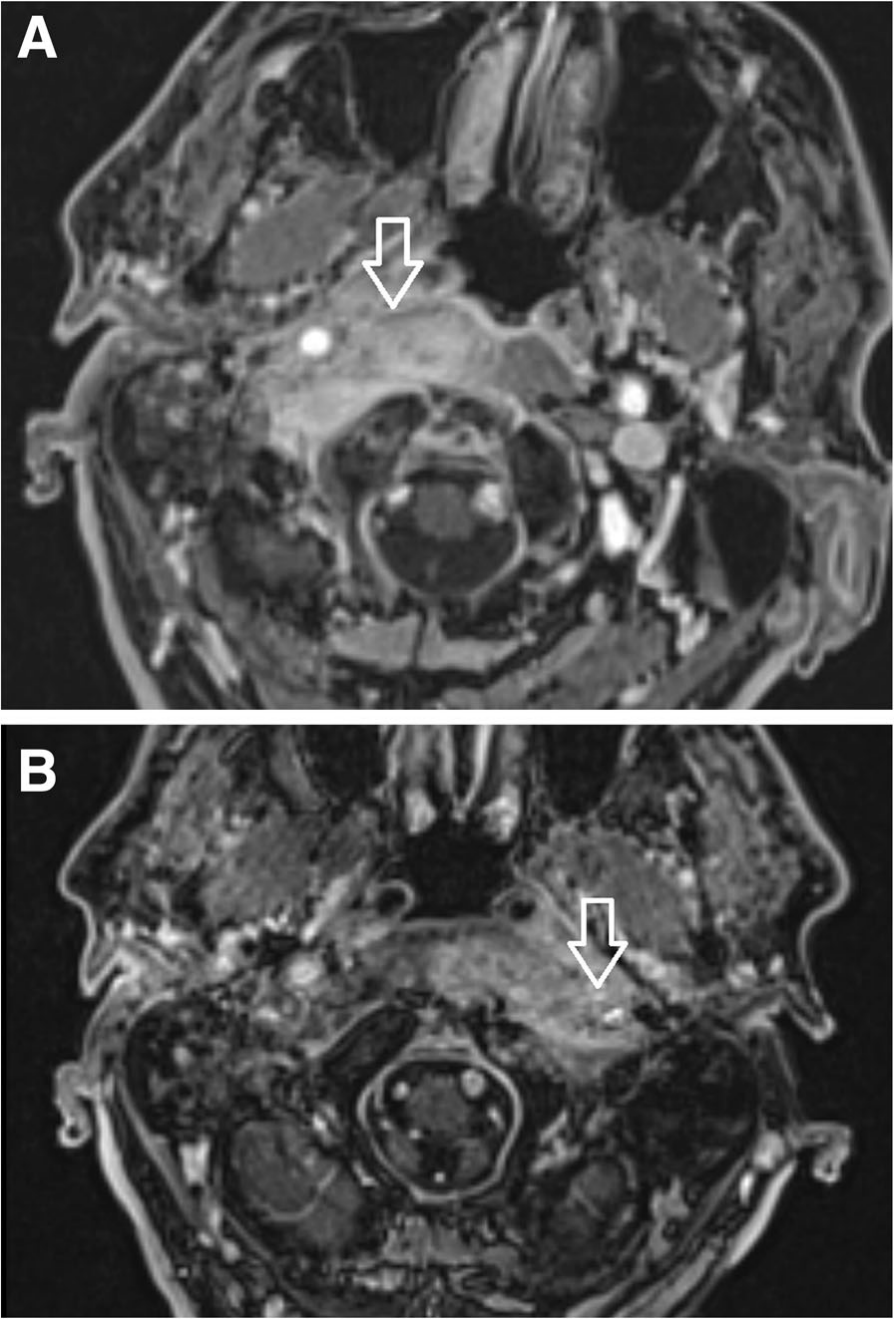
a Patient 1, 2 months after the first clinical symptoms: MRI of the skull base. Contrast-enhanced axial T1-weighted spin-echo image with fat saturation. Inflammatory changes of the right side soft tissues beneath the skull base, musculus longus capitis and musculus rectus capitis anterior (arrow). The swelling extends to the midline; the infection originates from the right external auditory canal. b Patient No. 1, 4 months following the first clinical symptoms: MRI of the skull base of the same patient. Contrast-enhanced axial T1-weighted spin-echo image with fat saturation. After antibiotic treatment: ceftazidime, ciprofloxacin, clindamycin, Oxacyllin. Inflammatory changes of the soft tissues below the skull base progressed to the left side (arrow), musculus longus capitis and musculus rectus capitis anterior. The swelling affects the retropharyngeal and retrostyloid part of the parapharyngeal space in the midline and progresses to the left side
